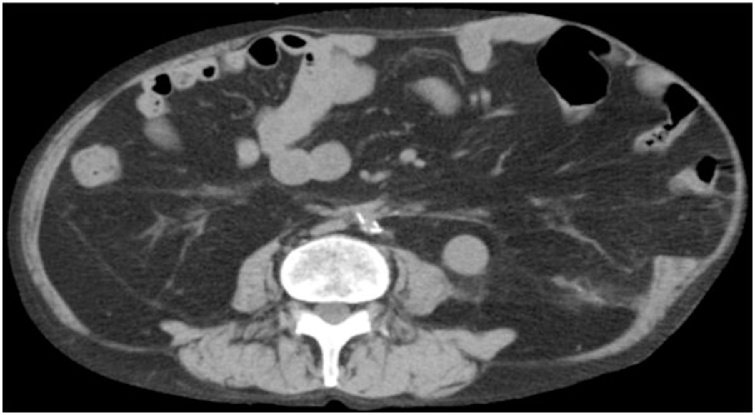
Computed tomography (CT) findings. CT revealed a 17 × 13 cm incisional hernia from the ninth rib to the left flank.
